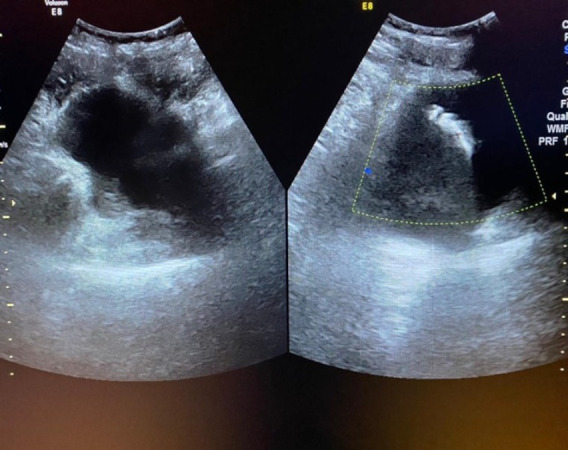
Ultrasound of bladder showing hernial mesh projecting into urinary bladder On Color Doppler, no significant blood flow seen
